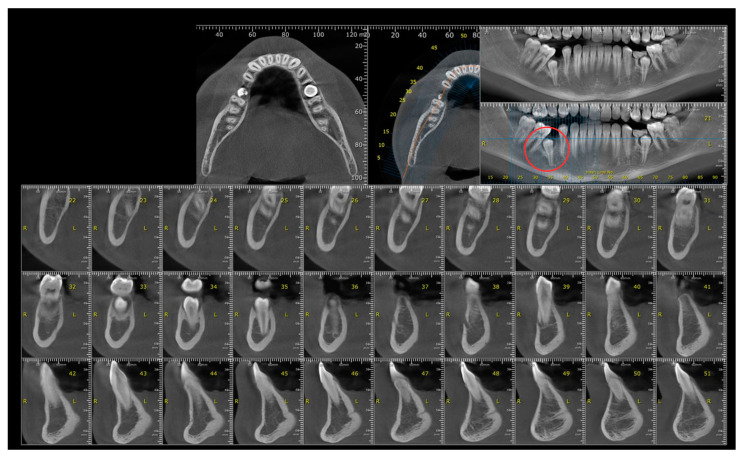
CBCT showing the proper eruption path for the second premolar.
